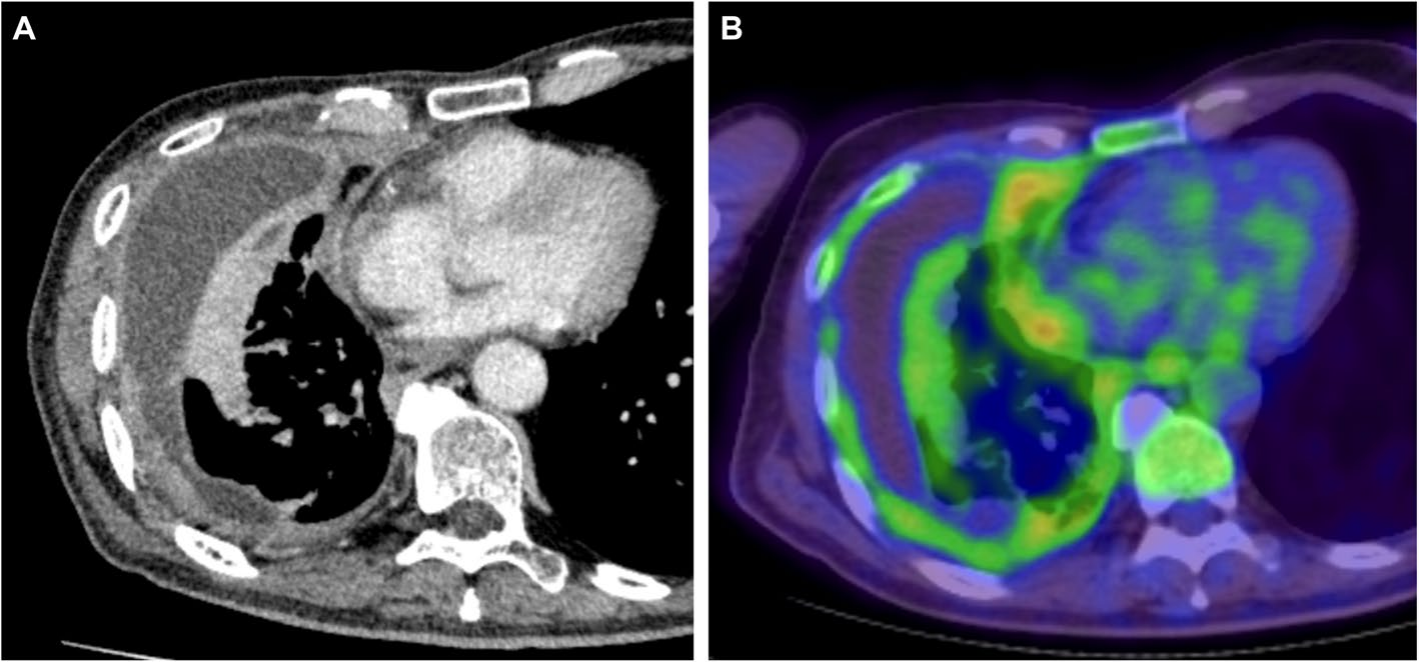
Preoperative chest computed tomography before chemotherapy. A Contrast-enhanced chest computed tomography reveals right pleural effusion with thickening of the pleura and B positron emission tomography computed tomography shows F-fluorodeoxyglucose uptake in the pleural
